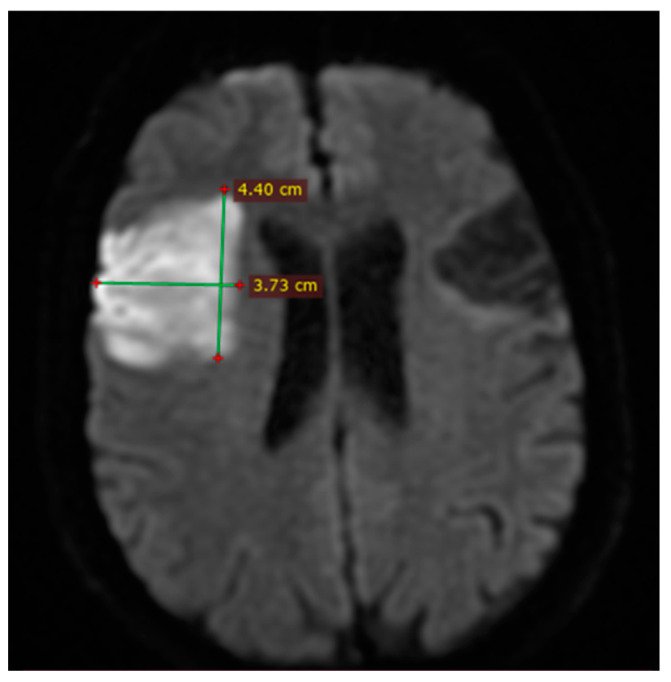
MRI DWI axial section. Example of measurement of stroke volume using ABC/2 formula.
